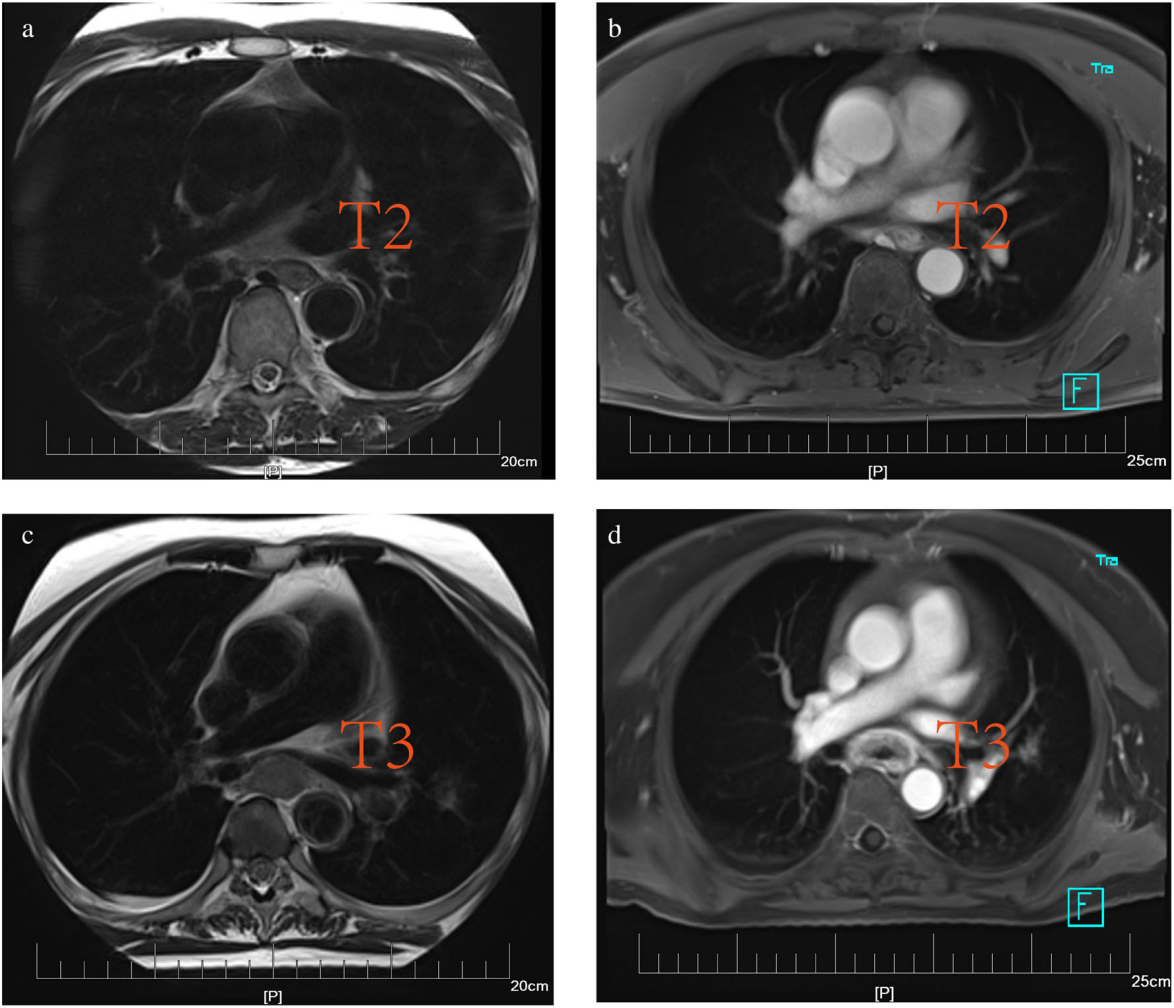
Magnetic resonance imaging (MRI) examination of patients with esophageal cancer ((a), (b) is the same patient, (c), (d) is the same patient). (a) T2 stage was diagnosed by T2WI sequence; (b) T2 was diagnosed under T1WI + c sequence; (c) T3 stage was diagnosed under T2WI sequence; (b) T3 stage was diagnosed under T1WI + c sequence.
